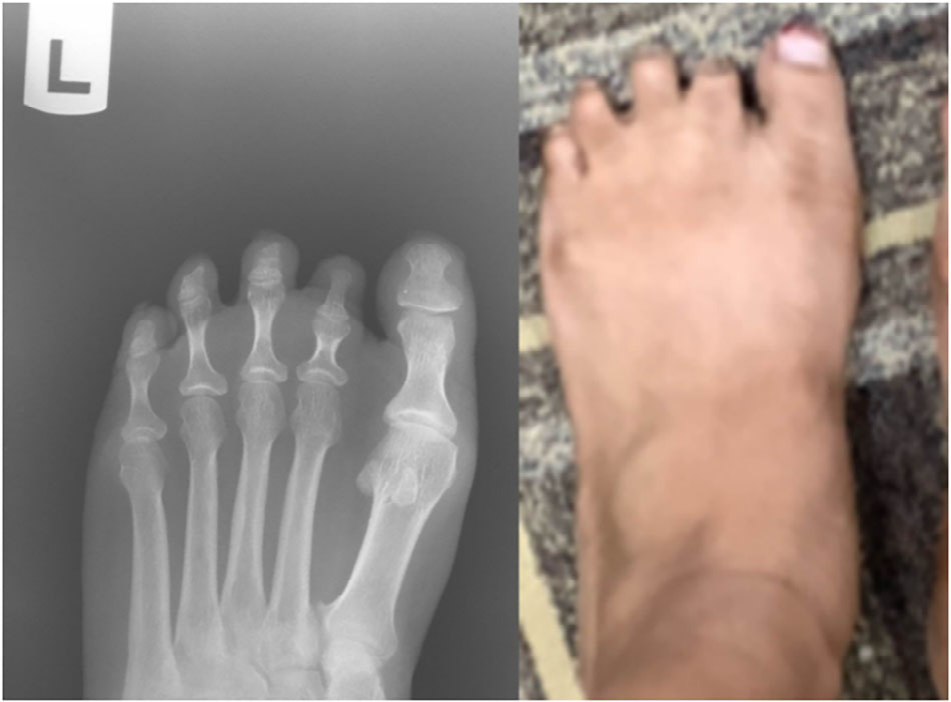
Imaging of a 34‐year‐old female exhibiting digit deformity in her left foot, shown in the radiograph and photograph.
